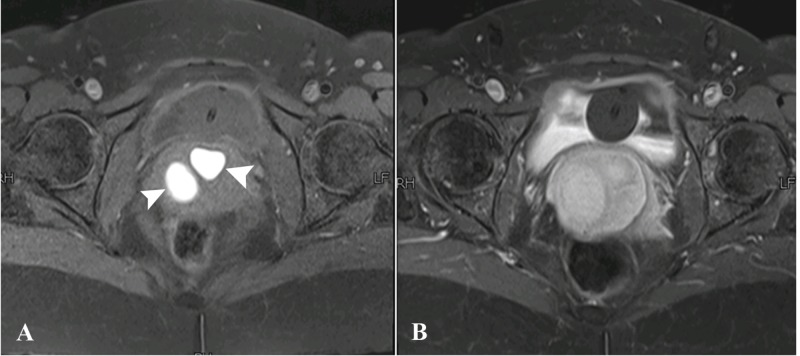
Magnetic resonance images - A) Axial pre- and B) Axial post-contrast T1-fat-sat sequences. A) Sections at the level of upper third of vagina showing rounded T1-hyperintense structures (arrowheads) representing hemorrhagic cystic spaces. B) These structures were not showing fat suppression.
